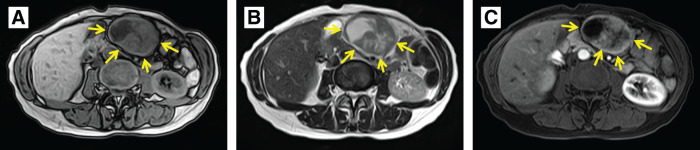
Preoperative MRI. (A) T1-weighted image. The tumor displayed low signal intensity (arrows). (B) T2-weighted image. The tumor showed a mixture of low and remarkably high signal intensities (arrows). (C) Contrast imaging demonstrated an early enhancement effect (arrows).
